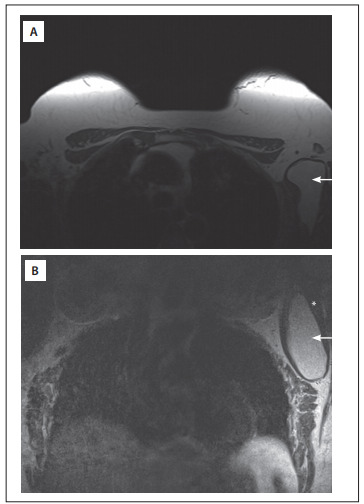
Magnetic resonance imaging. A: T1-weighted axial image; B: T2-weighted coronal image. Arrow, intramuscular lipoma. Asterisk, subscapularis muscle.
